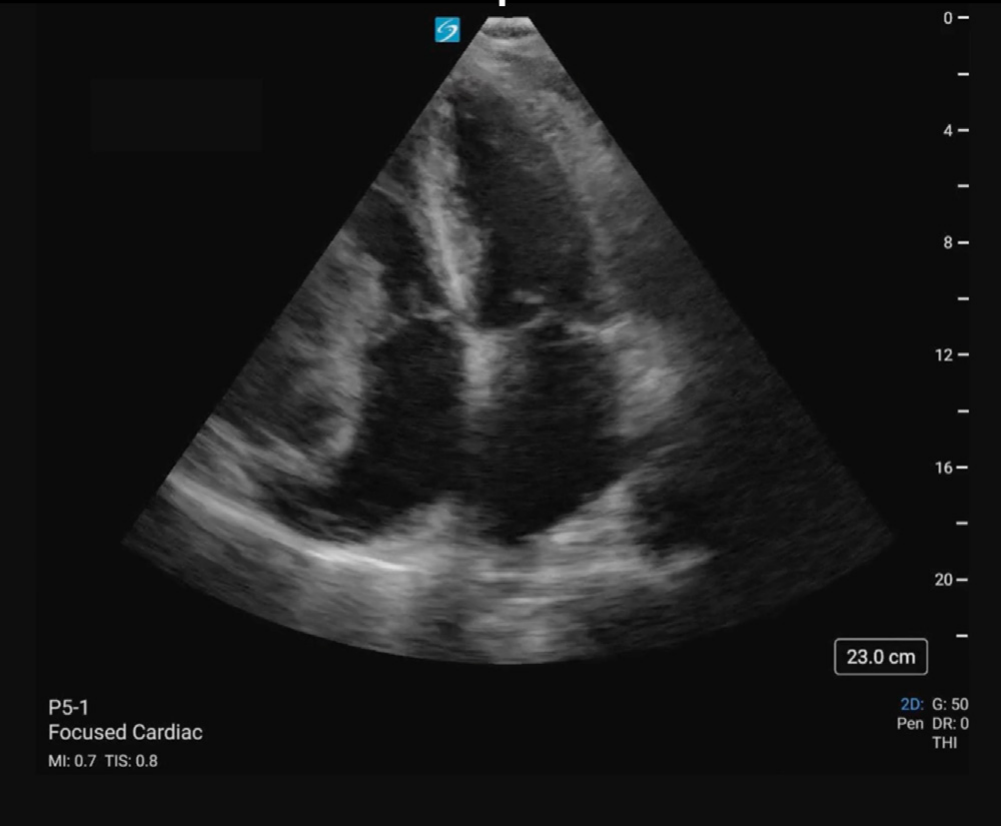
Apical 4-chamber view of the heart demonstrating a right heart mass with associated right ventricle free wall hypokinesis and small pericardial effusion.
